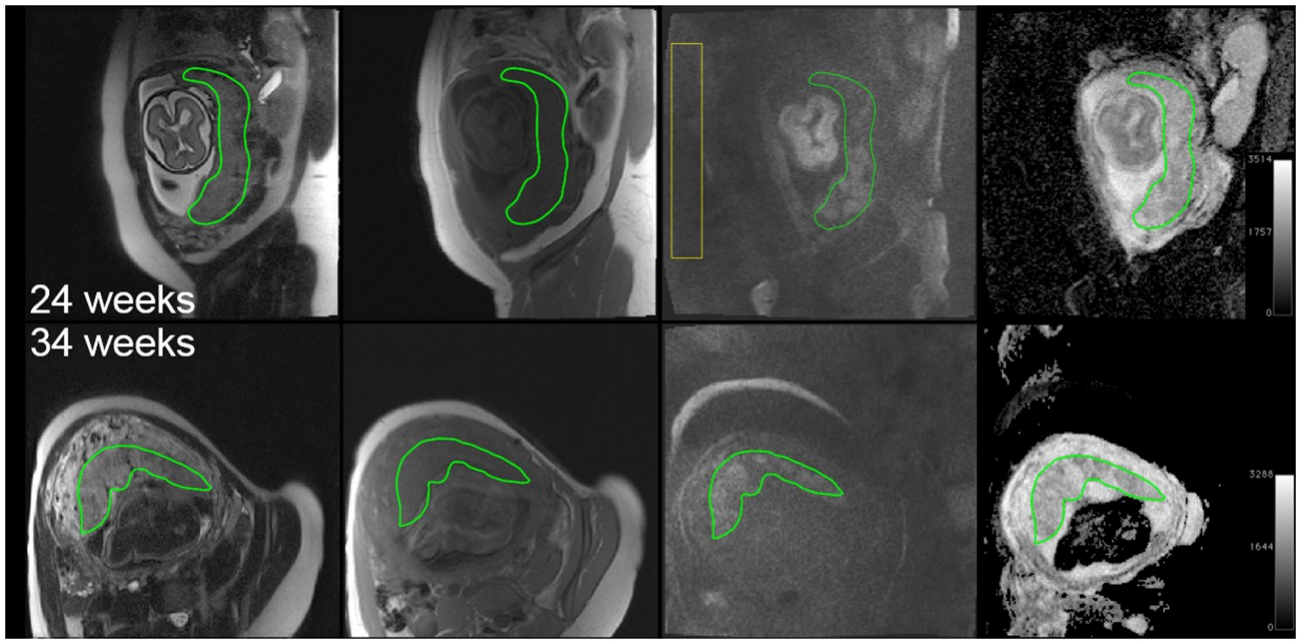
Left to Right: Representative T2W, T1W, DWI (b = 800 s/mm^2^), and ADC map of a 24-week (top row) and a 34-week (bottom row) gestation with their respective placental ROIs (green). For DW images SNR calculation, the image background noise ROI (yellow) is drawn outside of the tissue. The 24-week fetus was identified to have mild CNS ventriculomegaly; the 34-week fetus had normal MR findings.
